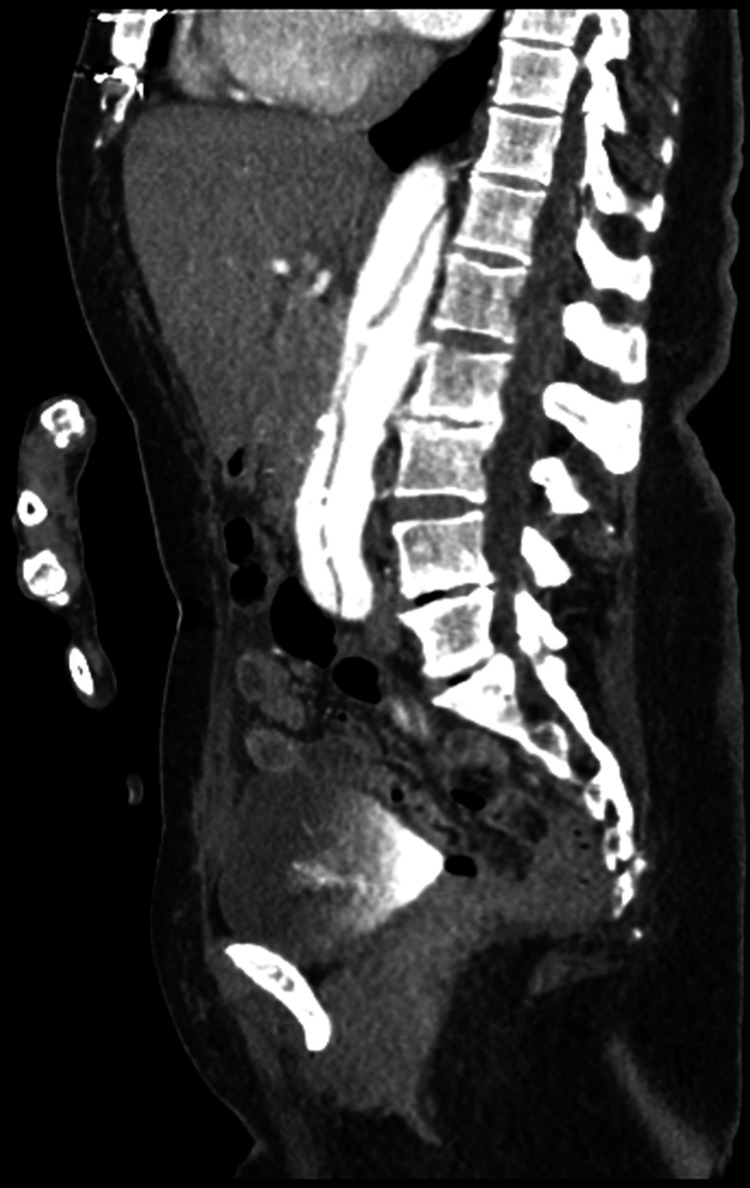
CTA of the abdomen and pelvis sagittal image with a repaired DeBakey type 1 dissection CTA: computed tomography angiography
